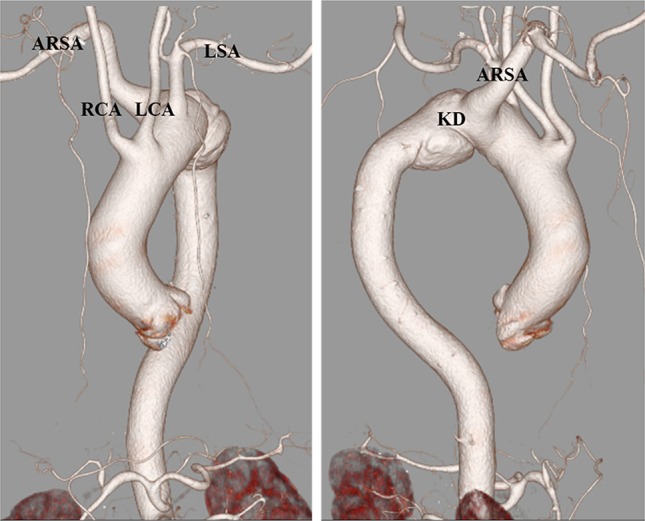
Preoperative computed tomography angiography. The Kommerell’s diverticulum with aberrant right subclavian artery. ARSA, aberrant left subclavian artery; KD, Kommerell’s diverticulum; LCA, left common carotid artery; RCA, right common carotid artery; LSA, right subclavian artery.
